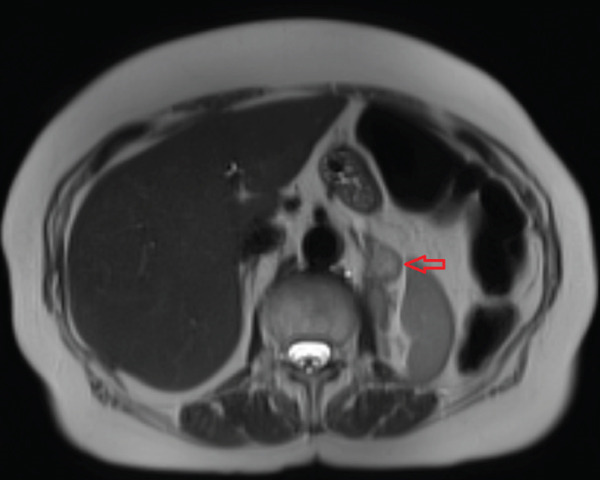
Axial abdominal MRI findings consistent with pancreatic metastases (red arrow).
